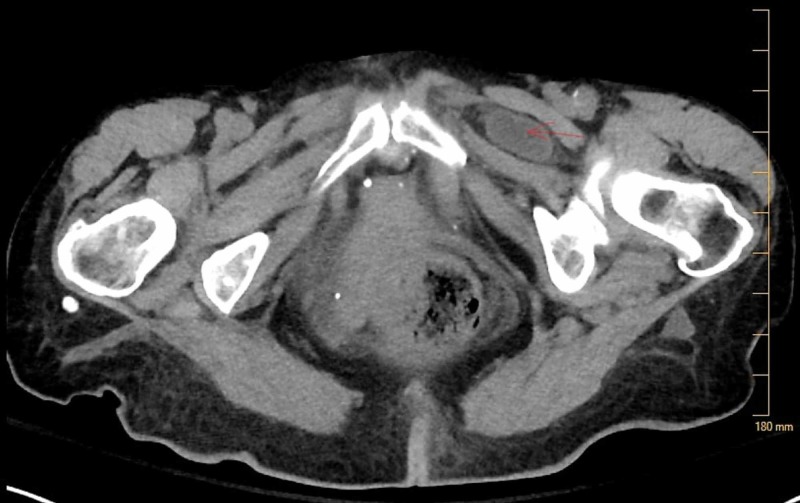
CT scan of the abdomen and pelvis showing the Left Obturator Hernia (Axial view).
